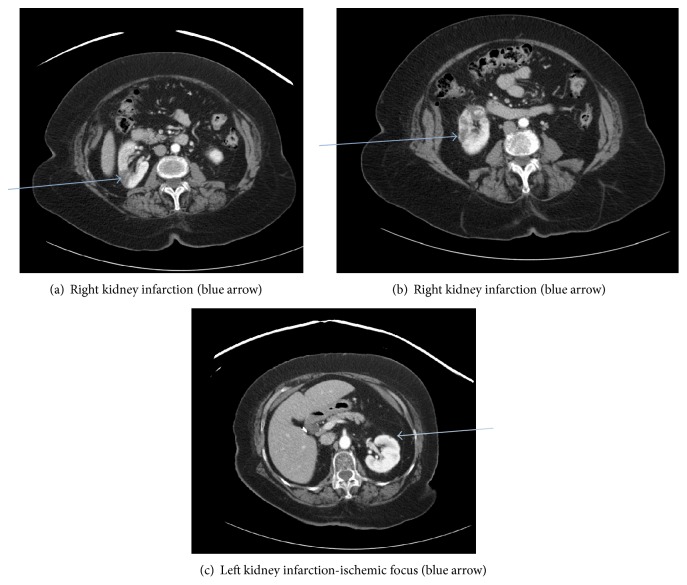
CT abdomen and pelvis with IV contrast. Findings: kidneys: both kidneys were not obstructed. A subcentimeter hypodense lesion is seen without calcification or septation in the upper pole of the left kidney. A wedge-shaped hypodense lesion is seen in the upper pole of the right kidney, which may represent a perfusion abnormality. Additional hypodense regions are seen in the mid pole of the right kidney, which may represent the sequela of a perfusion abnormality. Alternatively, these hypodense regions may represent lesions with soft tissue attenuation. In the lower pole of the right kidney, a 1.6 × 1.8 cm hypodense lesion is seen with internal enhancement.
